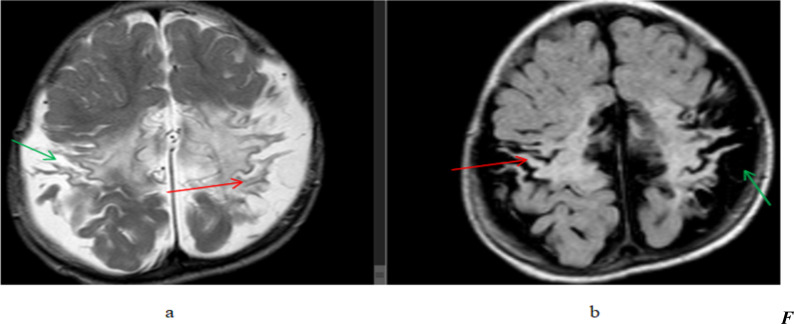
A 1-year-old male patient presented with developmental delay and a history of perinatal asphyxia, (a) T2W axial and (b) FLAIR axial, brain MR images showing bilateral parasagittal frontoparietal(perirolandic region) thinned gyri with hyperintense signal change(gliosis)(red arrows) and widening of sulci and subarachnoid CSF spaces(green arrows). Findings are consistent with the HIE sequela
